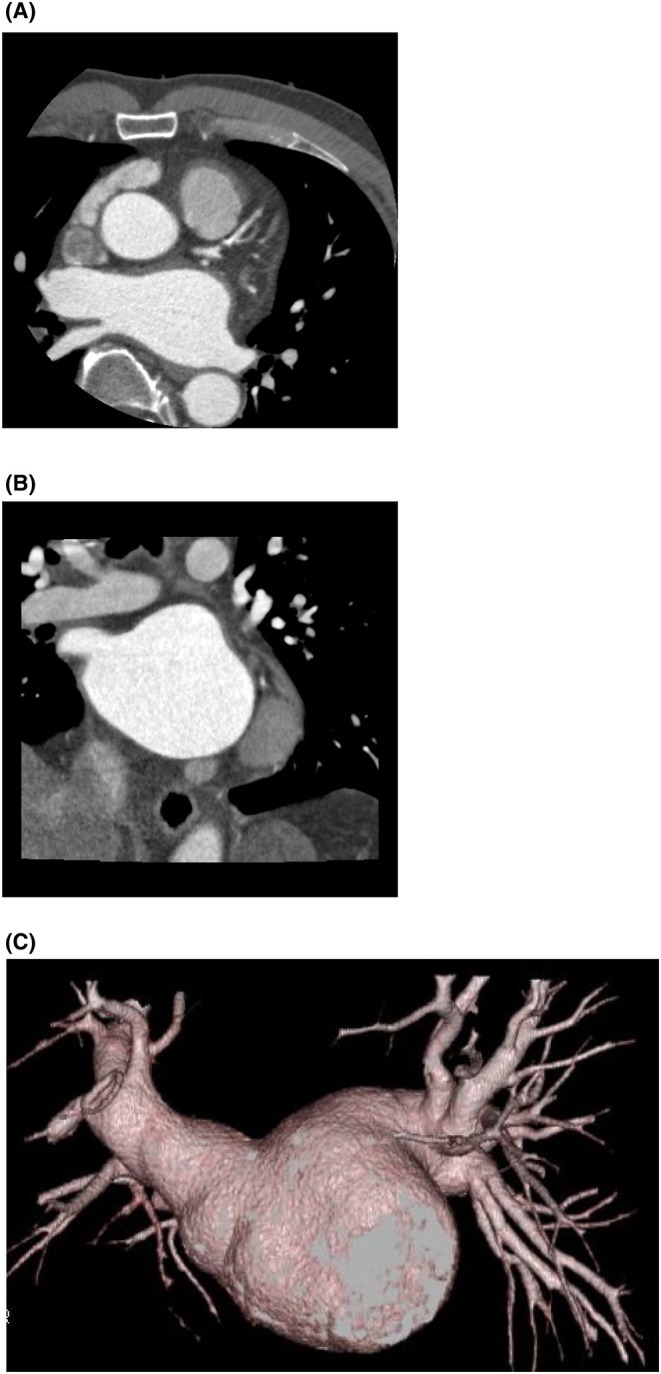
Preoperative contrast‐enhanced CT for ablation revealed congenital absence of the left atrial appendage (LAA). (A: Frontal view, B: Left lateral view).
